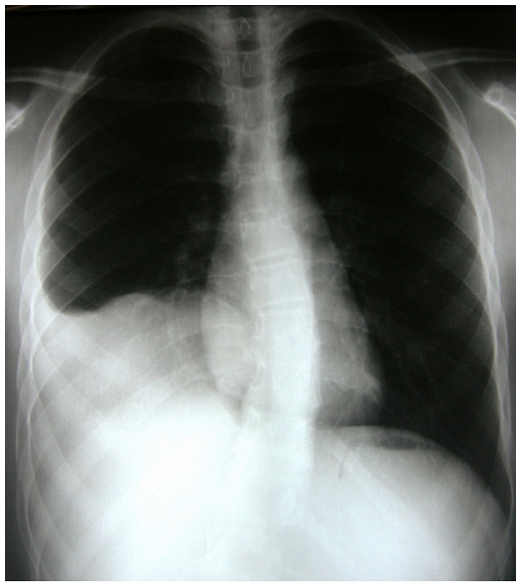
Radiographie thoracique de face montrant une opacité de tonalité
